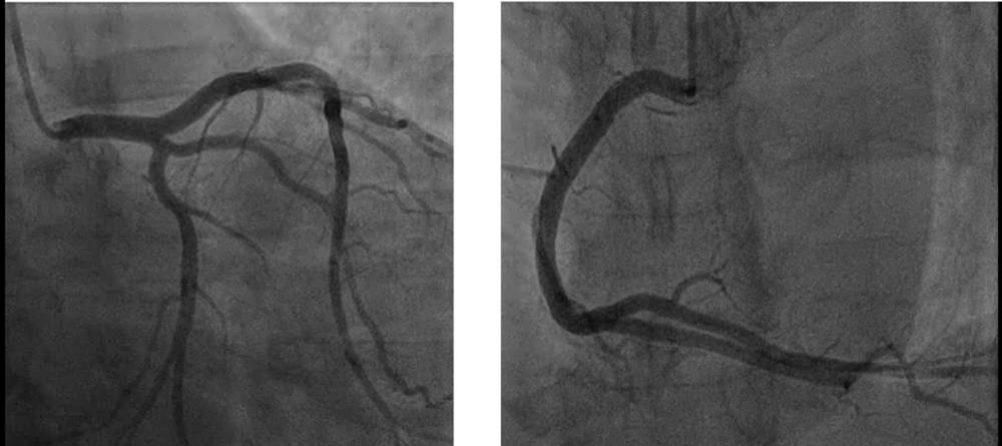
Representative images from invasive X-ray diagnostic coronary angiography showing unobstructed coronary arteries from 2014.
